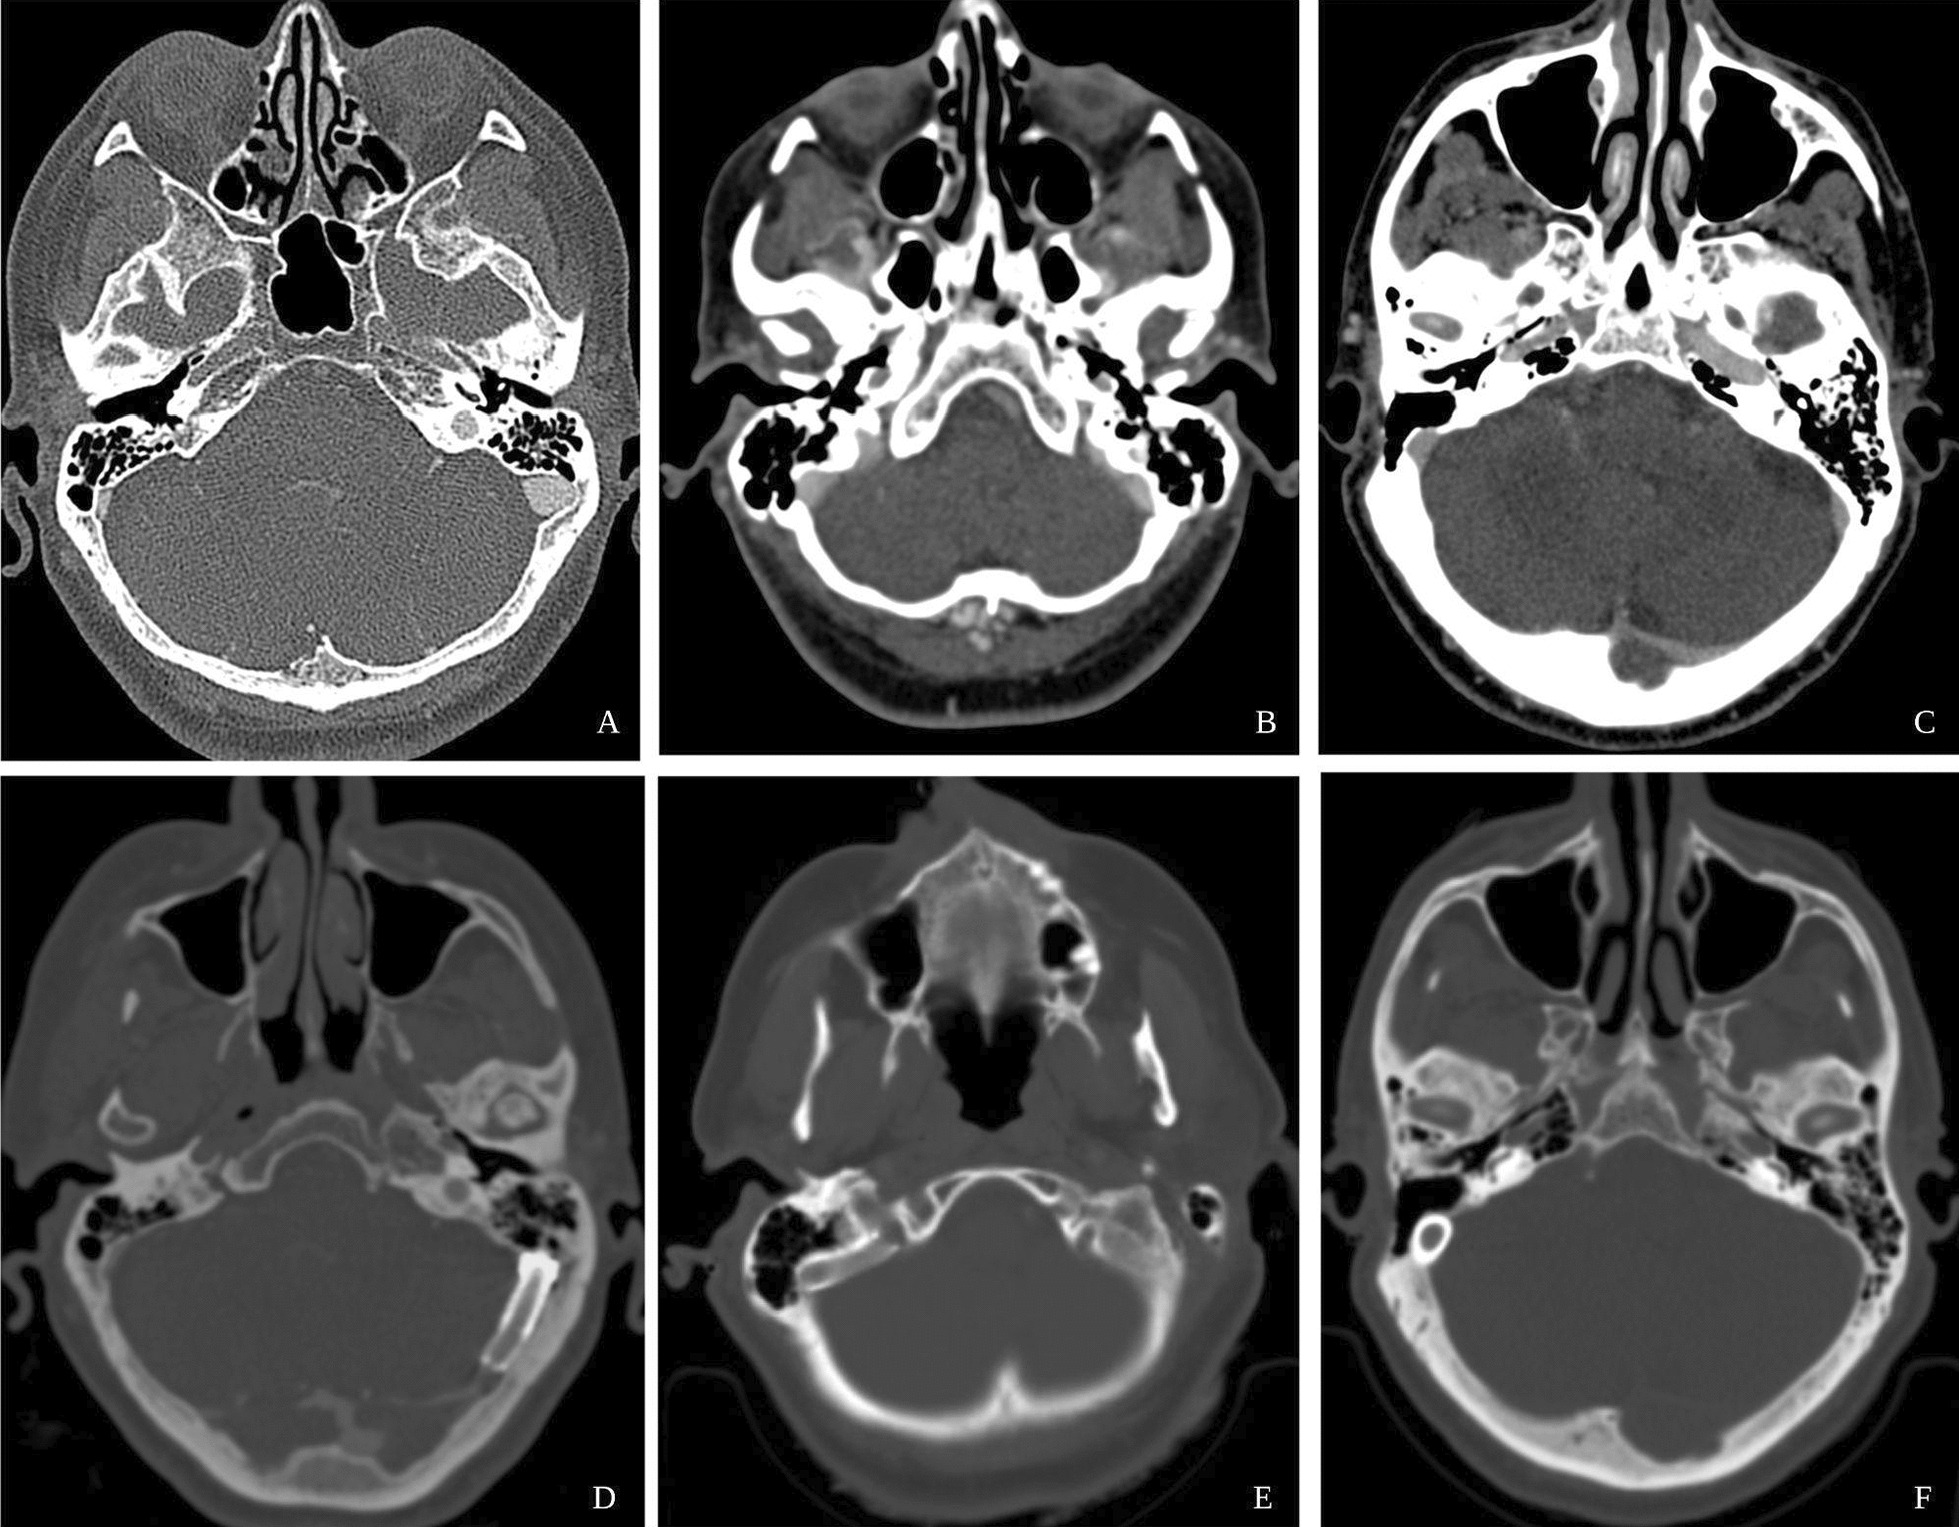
Preoperative and postoperative CTA images of patients 1, 2, and 3. A Patient 1 preoperative left SS dehiscence. B Patient 2 preoperative right SS dehiscence. C Patient 3: preoperative right SS dehiscence. D Patient 1 postoperative stent was deployed in the left SS. E Patient 2 postoperative stent was deployed in the right SS. F Patient 3 with a postoperative stent deployed in the right SS
